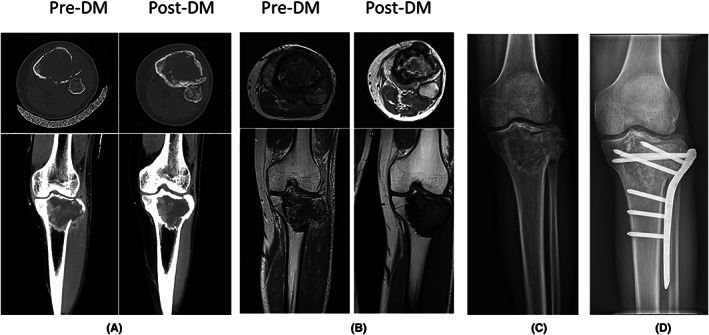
A 35‐year‐old men who was diagnosed with giant cell tumor (GCT) of proximal tibia and pathological fracture received preoperative denosumab treatment (six times). (A–C) CT, MRI, and x‐ray showed that the tumor was adjacent to the joint and involved the bone cortex. The peripheral sclerotic bone formation and the tumor margin became clear after denosumab treatment. (D) Curettage combined with internal fixation was performed, postoperative function was satisfactory without recurrence.
